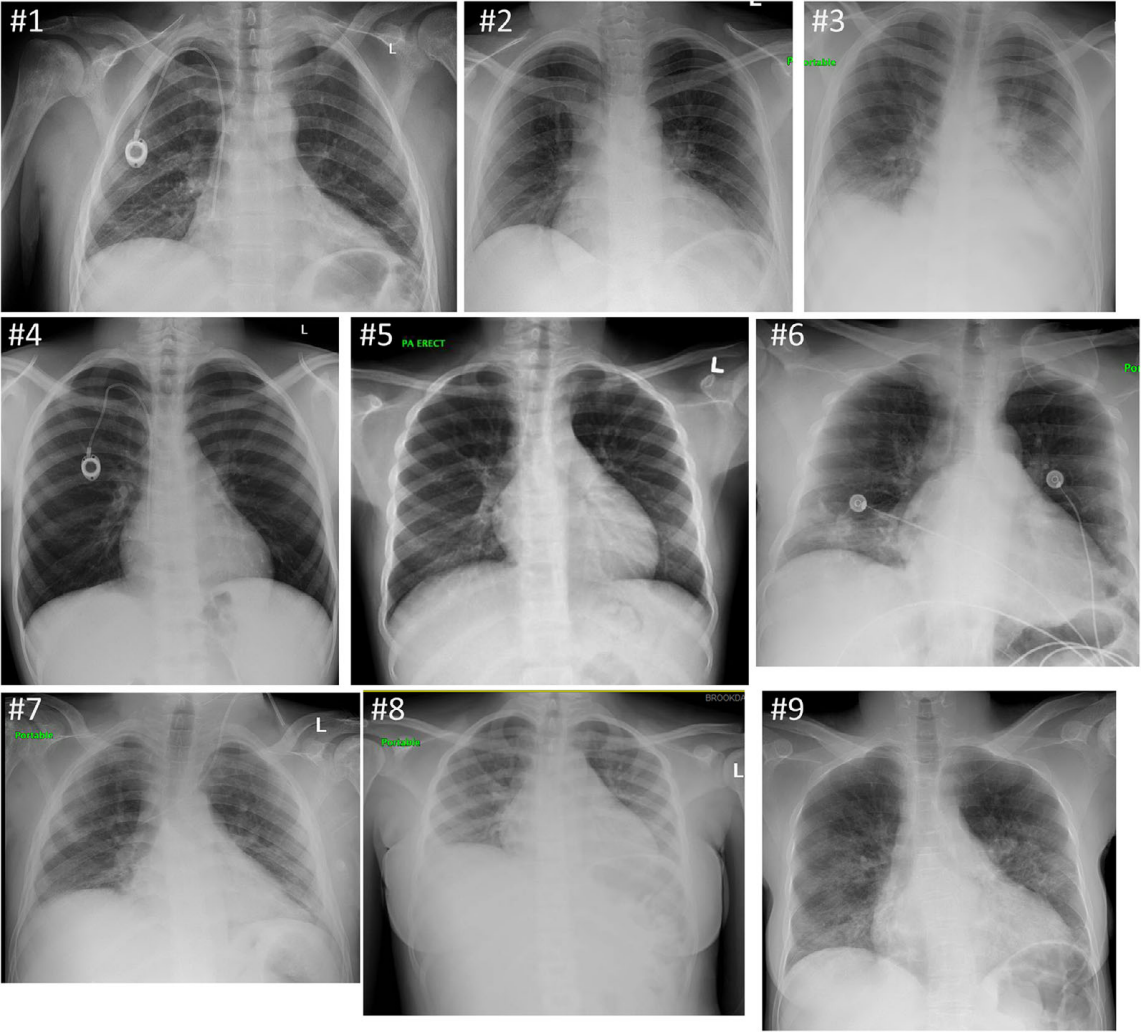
Chest radiograph of COVID‐19 patients with SCD
